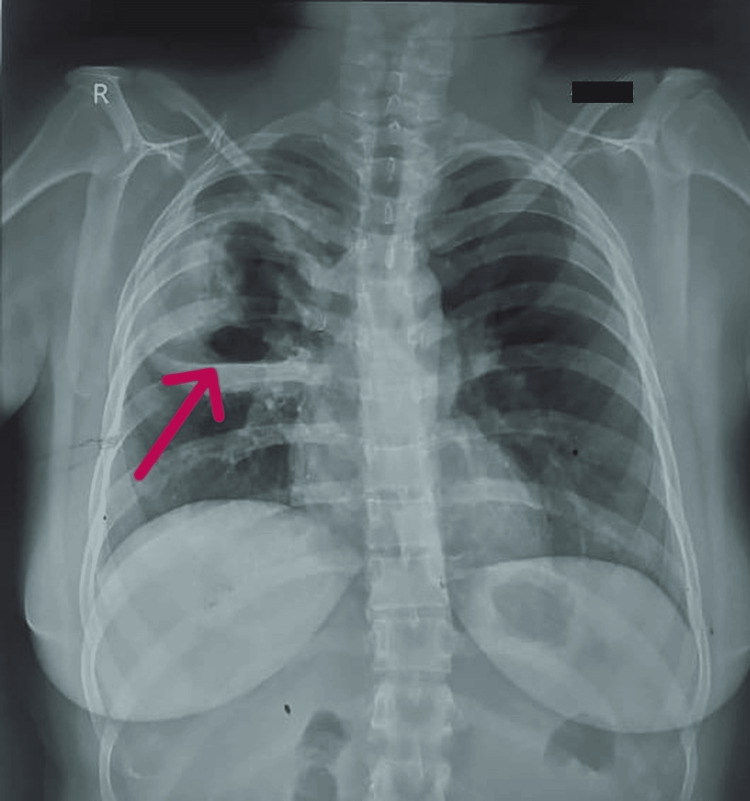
X-ray showing right lung thick-walled cavity lesion (arrow)
